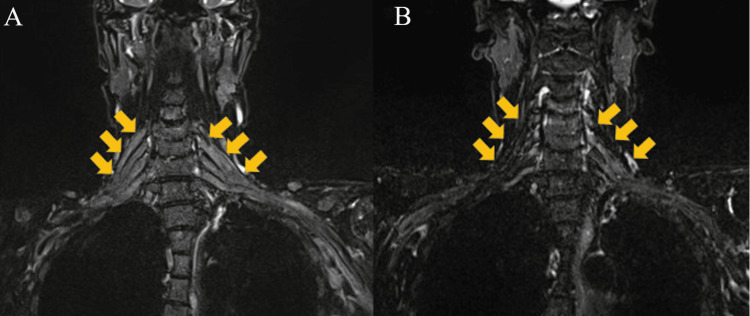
T2-weighted coronal magnetic resonance imaging (MRI) of the cervical area after diagnosis of Waldenström macroglobulinemia (MW)-associated peripheral neuropathy (PN) Yellow arrows indicate the brachial plexus. (A) Before treatment for PN and (B) 15 months after treatment with tirabrutinib.
